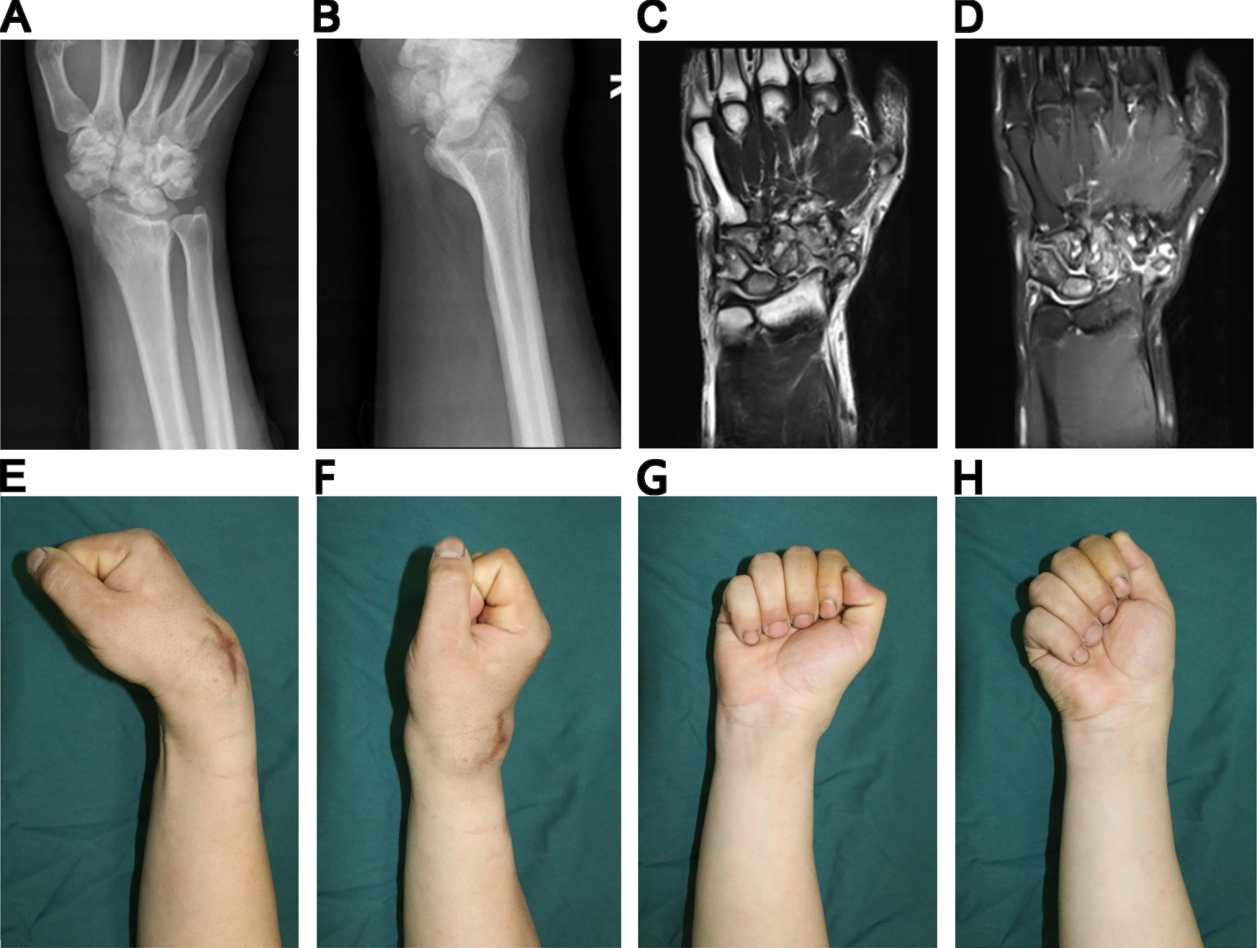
Pre-operative image and physical examinations. (A) Anteroposterior X-ray, (B) Lateral X-ray showing severe deterioration of the scaphoid. (C) & (D) MRI showing the soft tissue involvement as well. (E)–(H) Reduction in range of motion of the right wrist.
